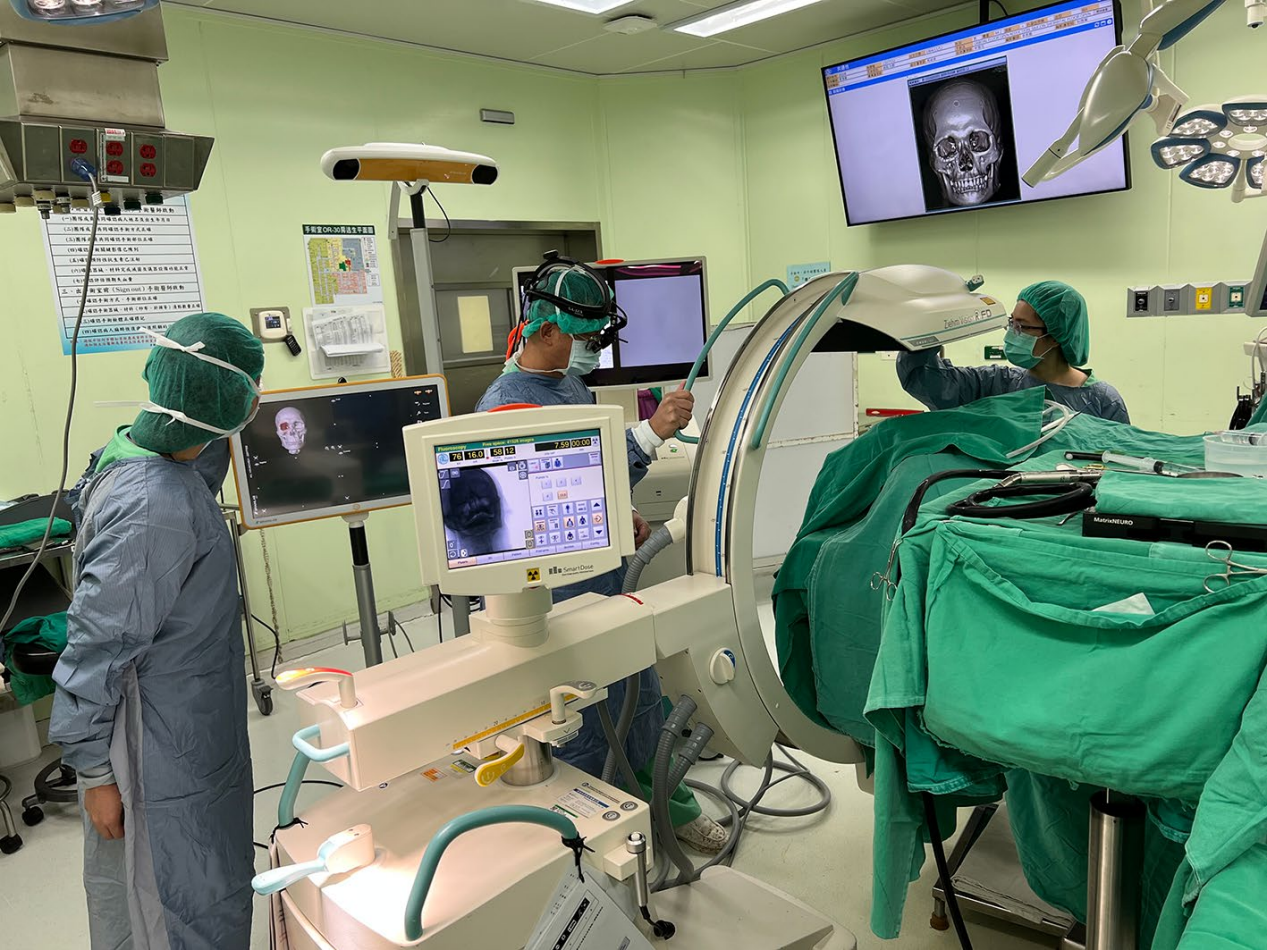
The setup of surgical navigation and intraoperative 3D C-arm computed tomography. The surgical navigation was used as a guide to determine the implant position. After plating, intraoperative 3D C-arm CT was performed to examine the adequacy of zygoma reduction and orbital reconstruction.
